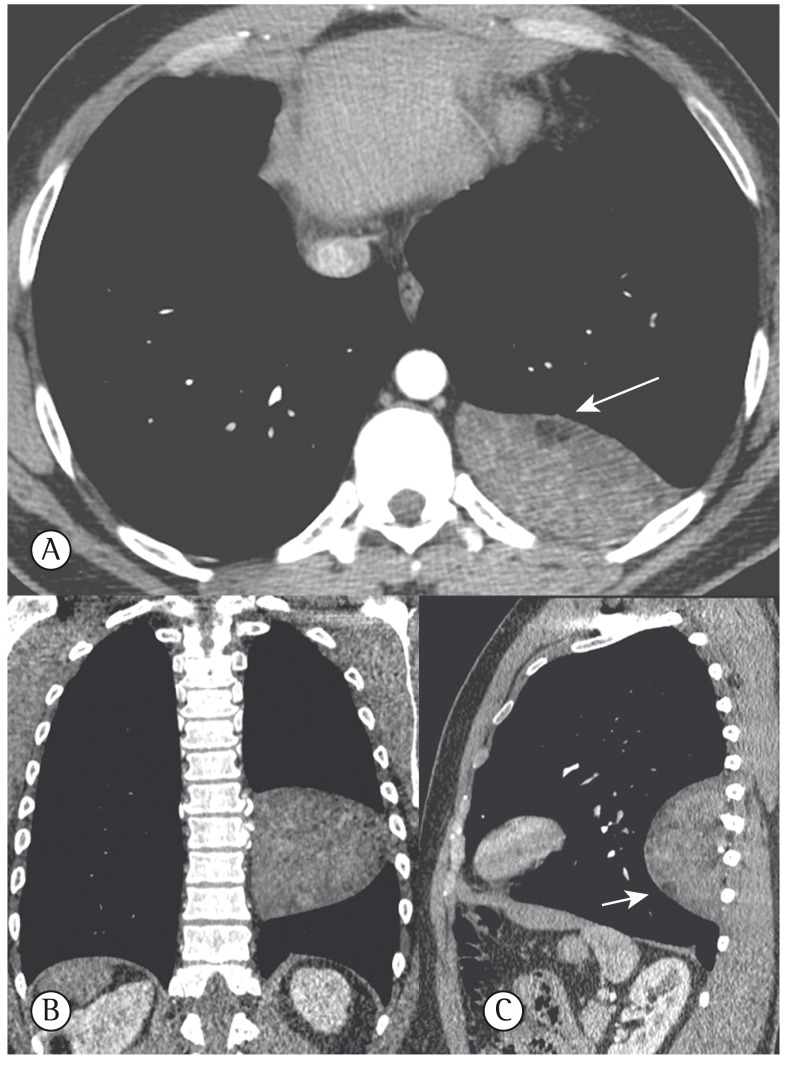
Chest CT reconstructions in the axial, coronal, and sagittal planes (A, B, and C, respectively), showing a heterogeneous pleural mass with areas of low-attenuation (fatty tissue, arrows) in the left lower hemithorax.
